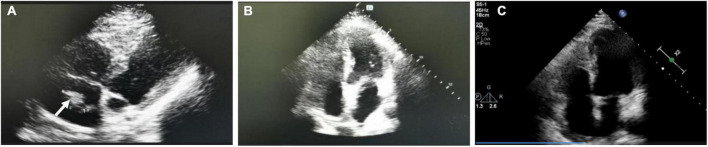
Echocardiography during the second hospitalization and follow-up period. (A) Right atrial mass became less after a week of anticoagulation. (B) Right atrial mass disappeared completely after 2 weeks of bridging anticoagulant therapy. (C) Echocardiography showed no right atrial thrombus (Follow-up period).
